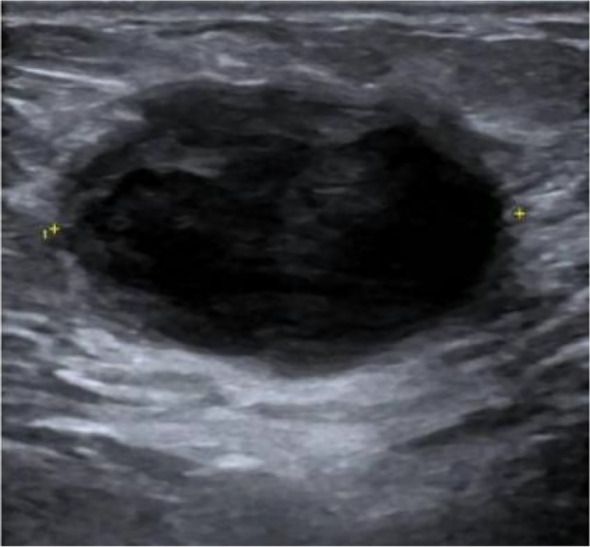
Ultrasound findings of Case 1 (February 2025). Ultrasound demonstrates a well-circumscribed cystic and solid mass (72mm×52mm×65mm) in the outer right breast. No definite internal vascularity is detected on Doppler imaging, consistent with a BI-RADS category 4A assessment.
